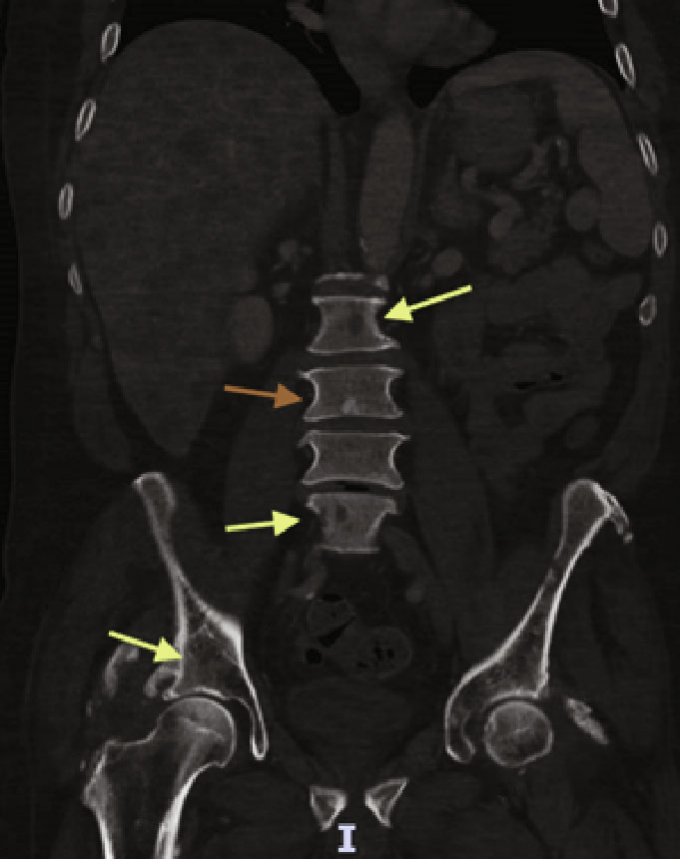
Coronal sections obtained from computed tomography of the abdomen and pelvis show sclerotic (orange arrow) and lucent foci (yellow arrows) within the iliac bones and vertebral bodies.
